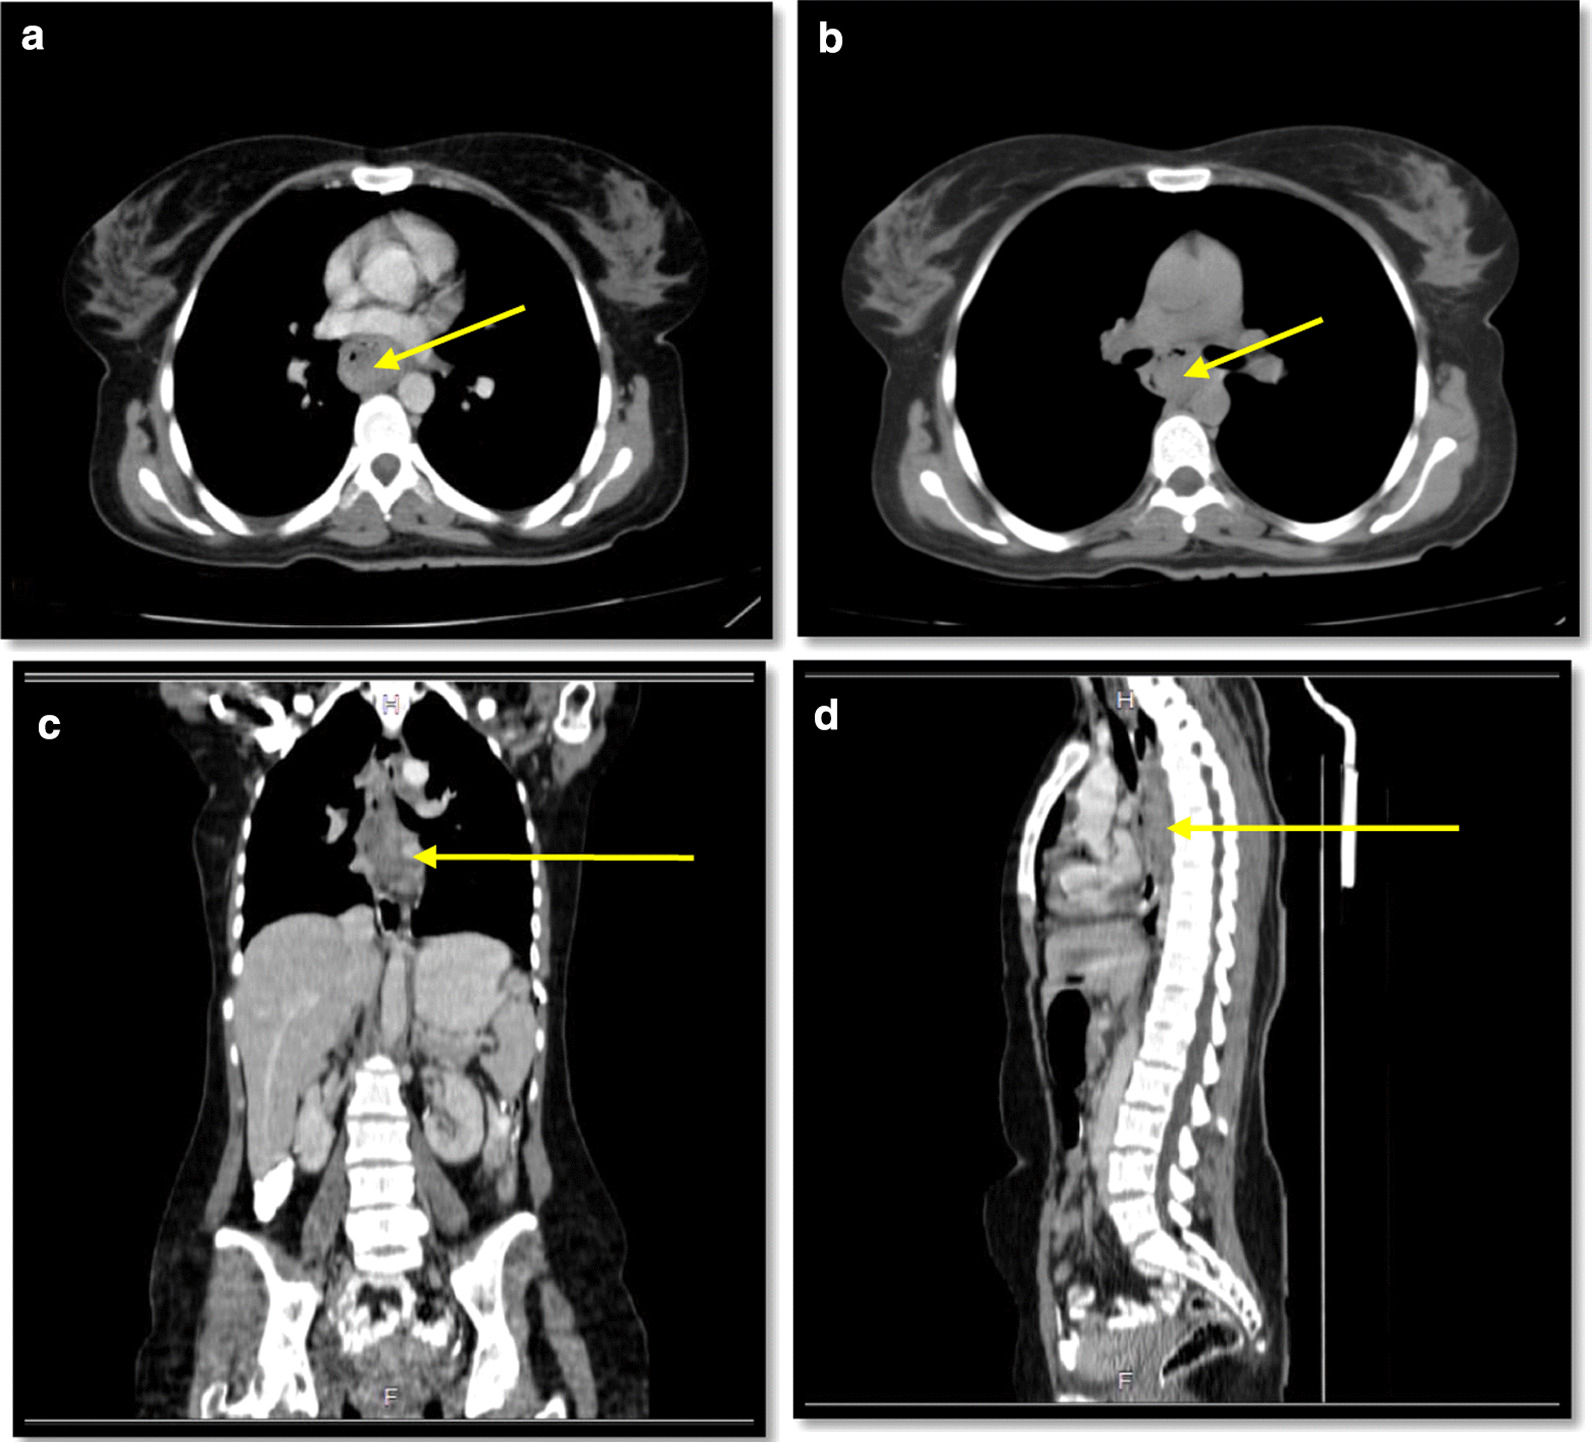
Spiral chest CT scan with and IV contrast (yellow arrows show the tumor); a axial view with IV contrast; b axial view without contrast; c coronal view with IV contrast; d sagittal View with IV contrast
